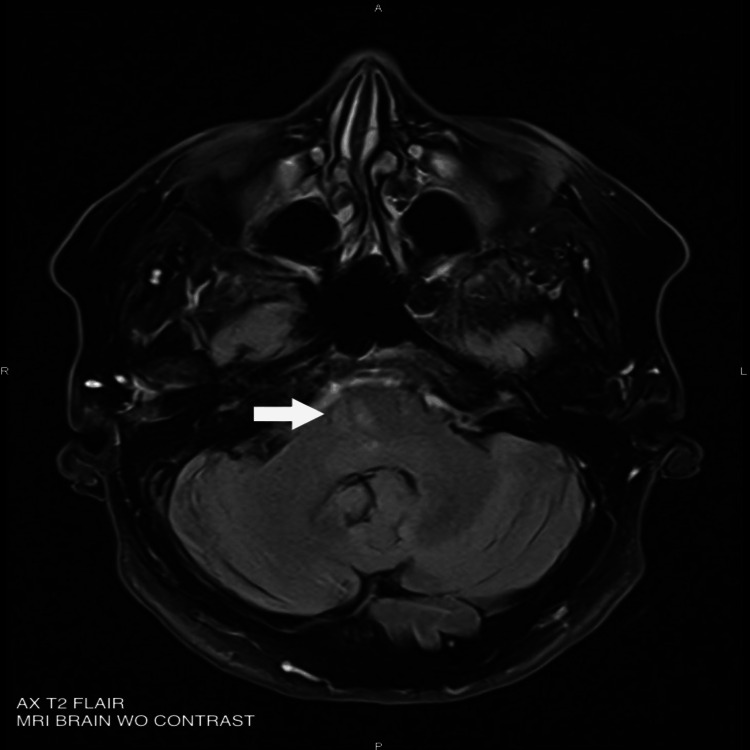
Brain MRI of the patient White arrow: Hyperintense lesion on FLAIR sequence in the right inferolateral pons; FLAIR: Fluid-attenuated inversion recovery; AX: Axial; WO: Without
